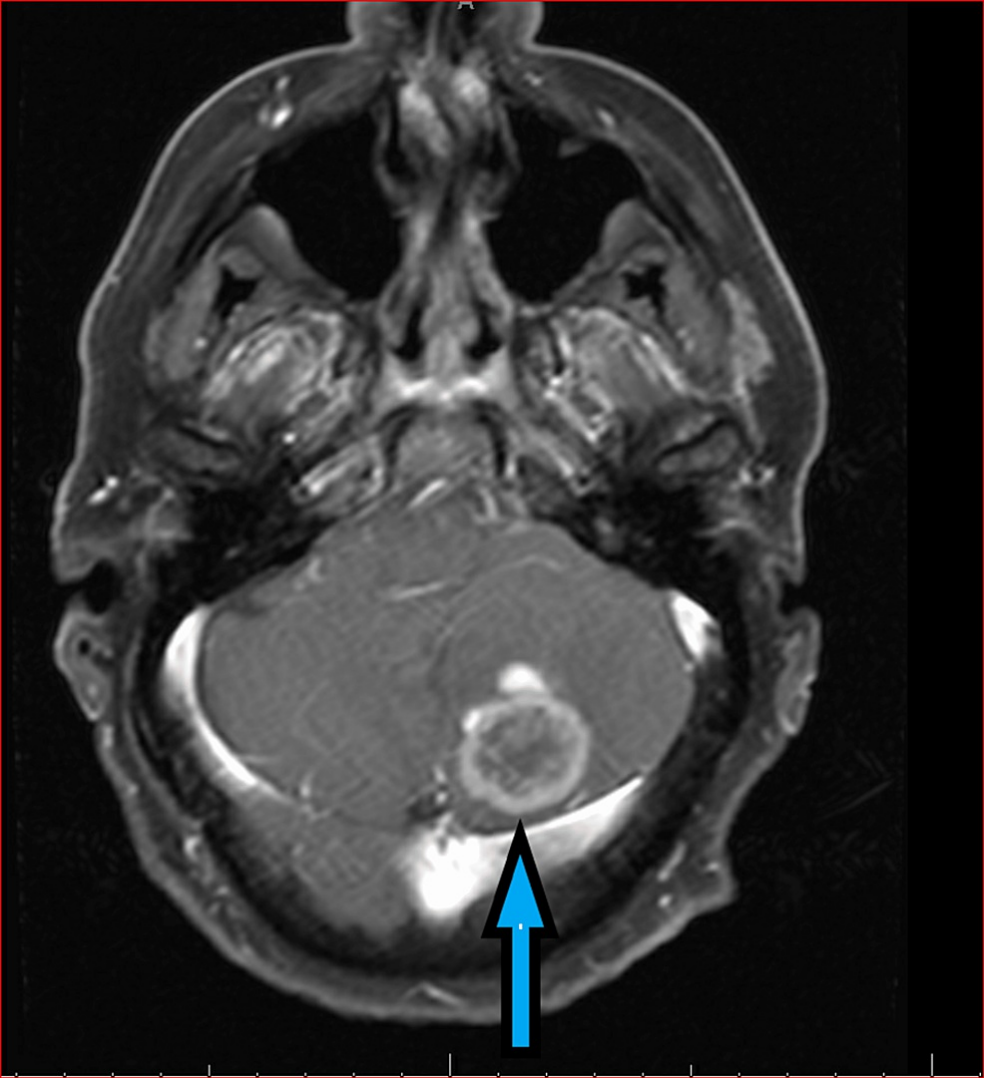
MRI brain image The image shows a peripherally enhancing mass in the left cerebellum measuring 3.2 x 2.9 x 2.9 cm.
